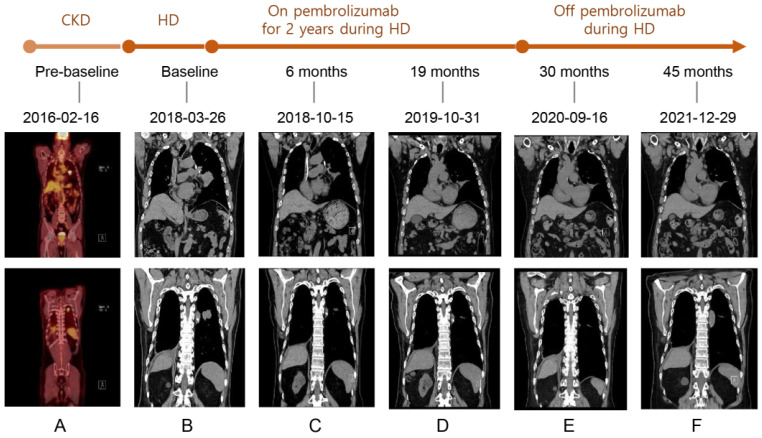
[F-18]FDG PET/CT scan and serial CT scans of the chest before and after pembrolizumab administration. (A) The [F-18]PET/CT scan on 16 February 2016 showed [F-18]FDG uptake in metastatic nodules in left upper lobe and left lower lobe. (B–F) The CT scans serially showed decreased extent of metastases in left lower lobe and left lower lobe from 26 March 2018 to 29 December 2021.
